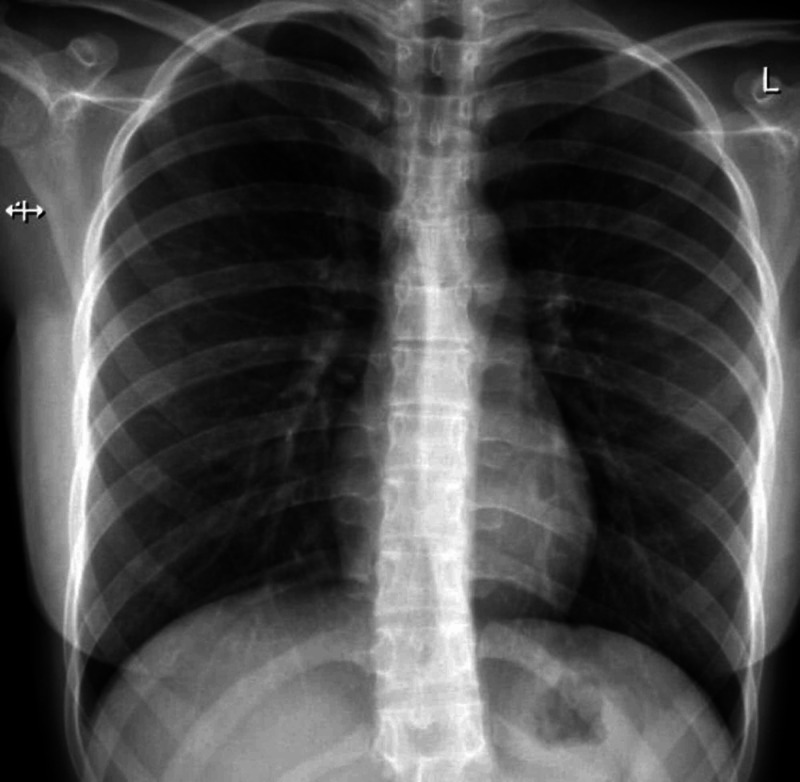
Chest Xray was unremarkable.
